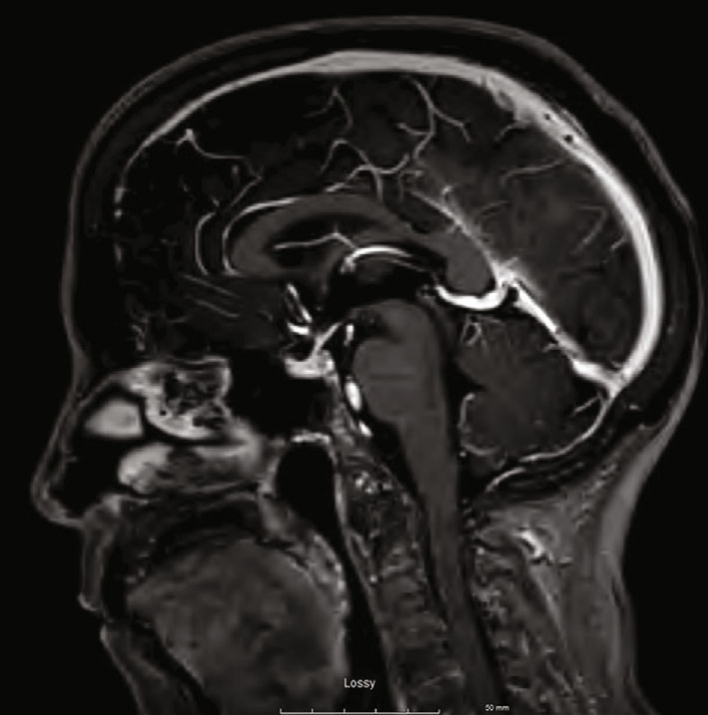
Brain MRI done before the treatment was started. No evidence of metastatic disease. Hypophysis appears normal.
